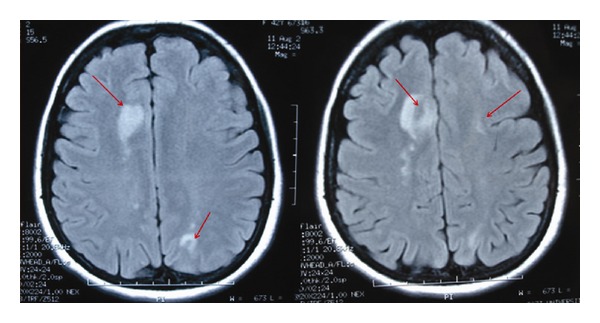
Brain MRI (FLAIR scans) with subacute ischemic lesions in left frontal and bilateral parietal regions.
